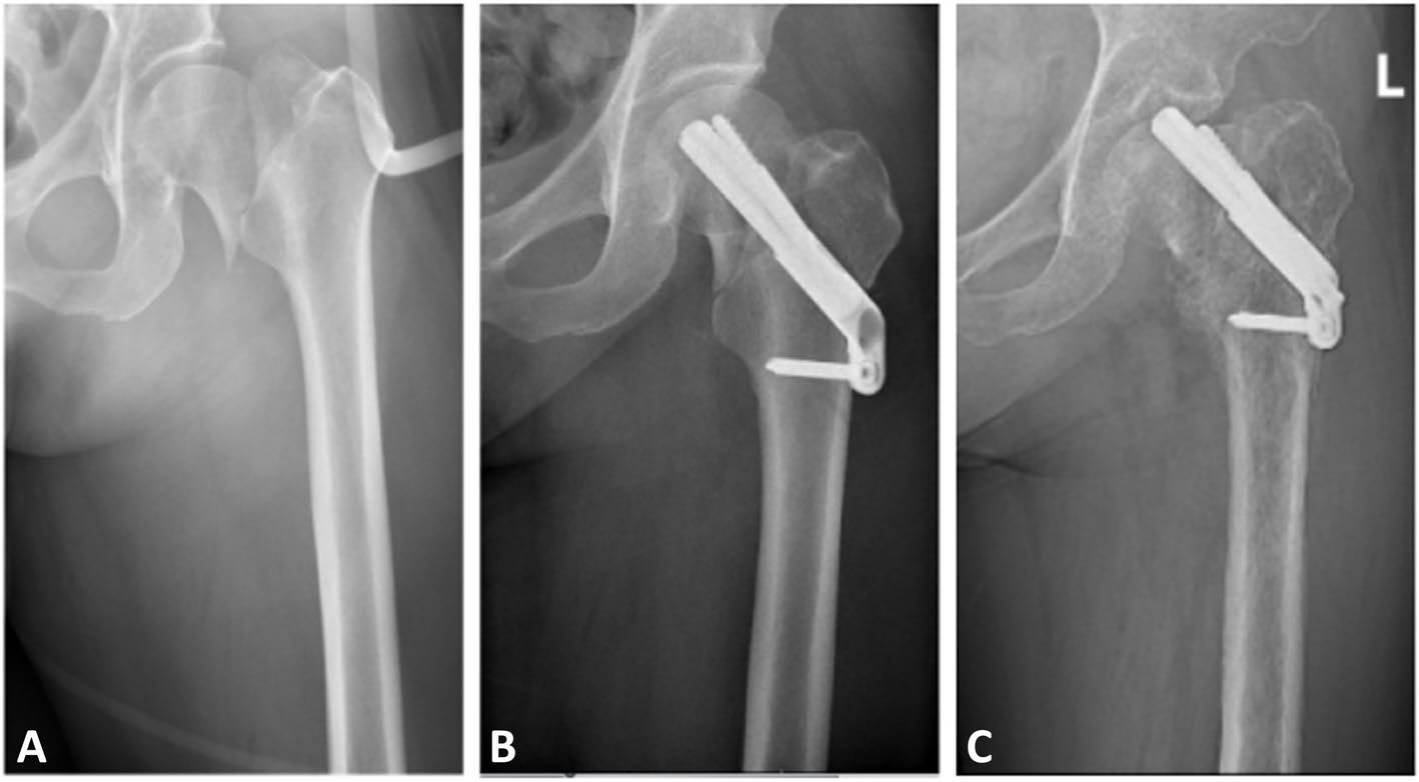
Preoperative anteroposterior radiograph of a left femoral neck fracture (Garden IV) in a 57-year-old woman (A). Anteroposterior radiograph at 2-month follow-up showing excellent healing and no evidence of femoral head necrosis (B). Anteroposterior radiograph at 6-month postoperatively showing screw-out and femoral head necrosis (C)
